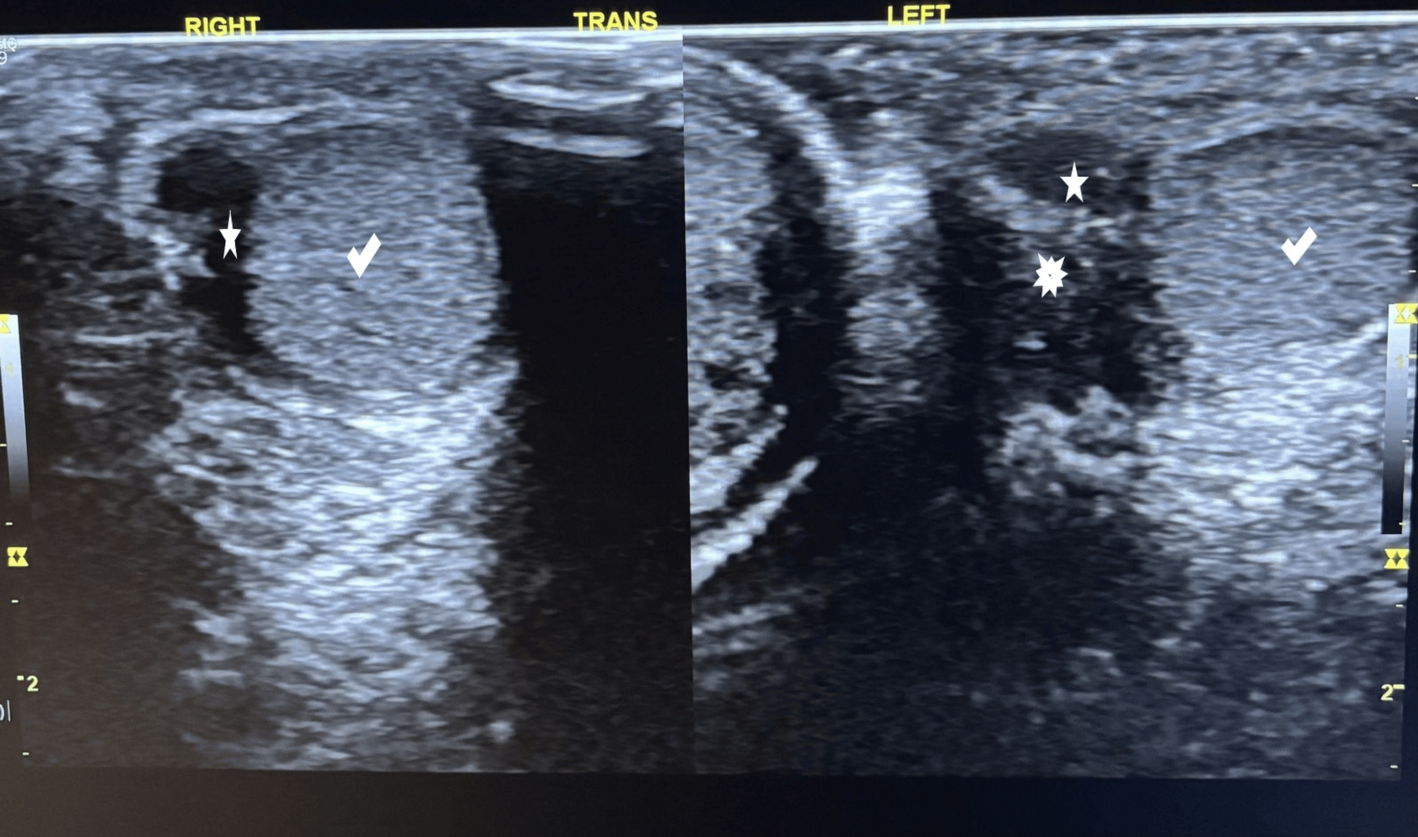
Follow-up scrotal ultrasound at the second postoperative year (age 3.5 years; transverse section) Transverse scrotal ultrasound images showing both testes (right and left check marks), in normal intrascrotal position, with smooth contours and homogeneous echotexture of the testicular parenchyma. The right epididymis (right five-point star) appears hypoechoic and is attached to the testis. The left epididymis (left five-point star) appears relatively posterior with focal hyperechoic areas (multipoint star), possibly representing fibromuscular layers
